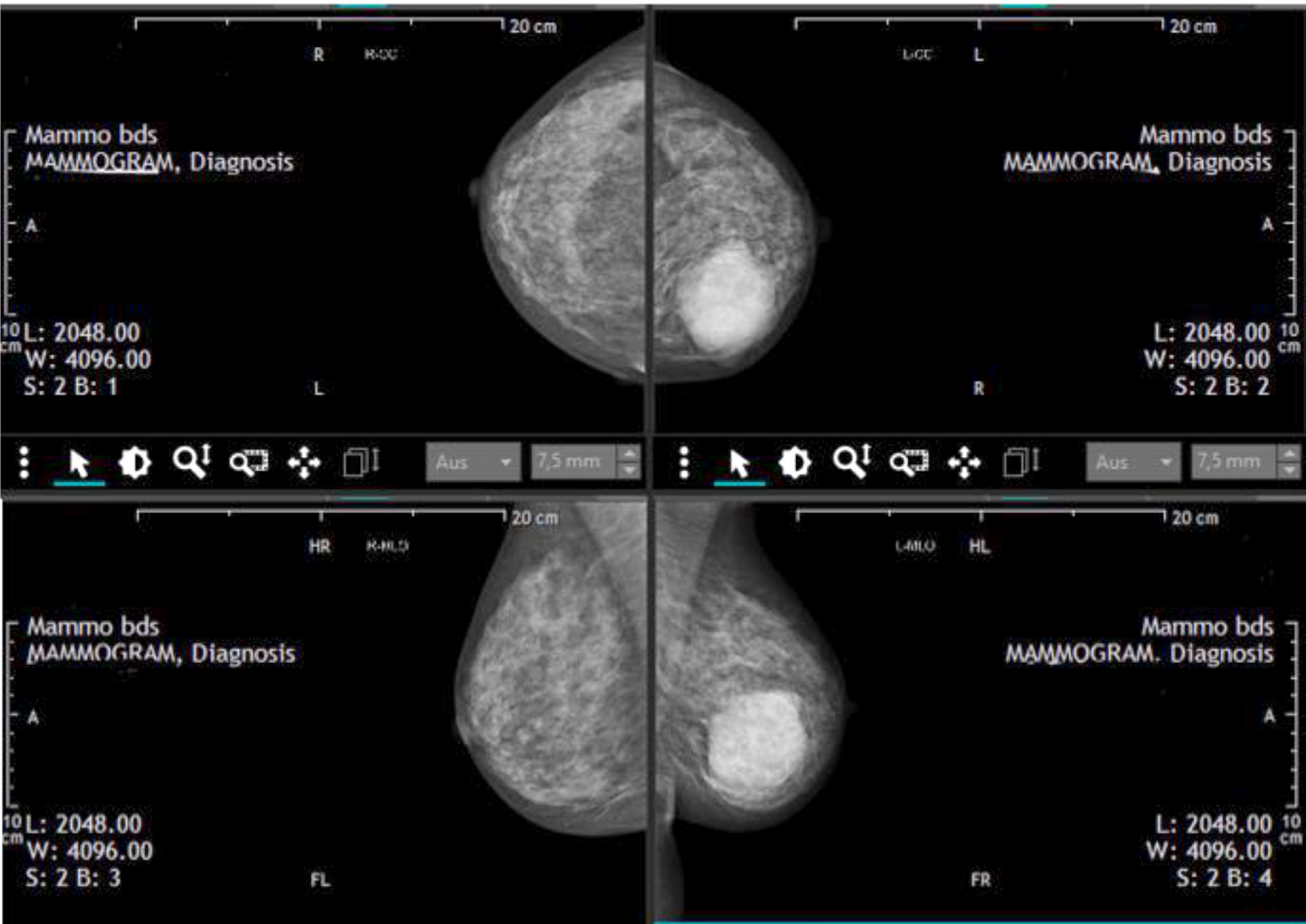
Ultrasonography results. Case 1
